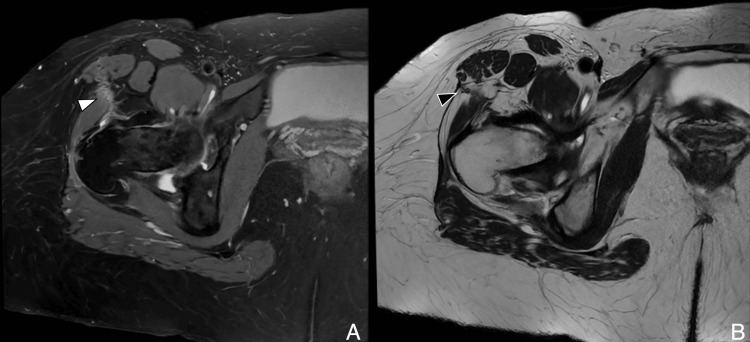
Outside-in arthroscopy (portal tract evolution) (A) Early postoperative axial PDFS image shows anterolateral portal tract as high signal through TFL and gluteus medius, extending to the joint capsule (white arrowhead). (B) Late postoperative axial T2-weighted MRI shows tract replaced by hypointense scar tissue (black arrowhead). Image Credit: Juan Pablo Muñoz, corresponding author PDFS: proton density fat-saturated; TFL: tensor fasciae latae
